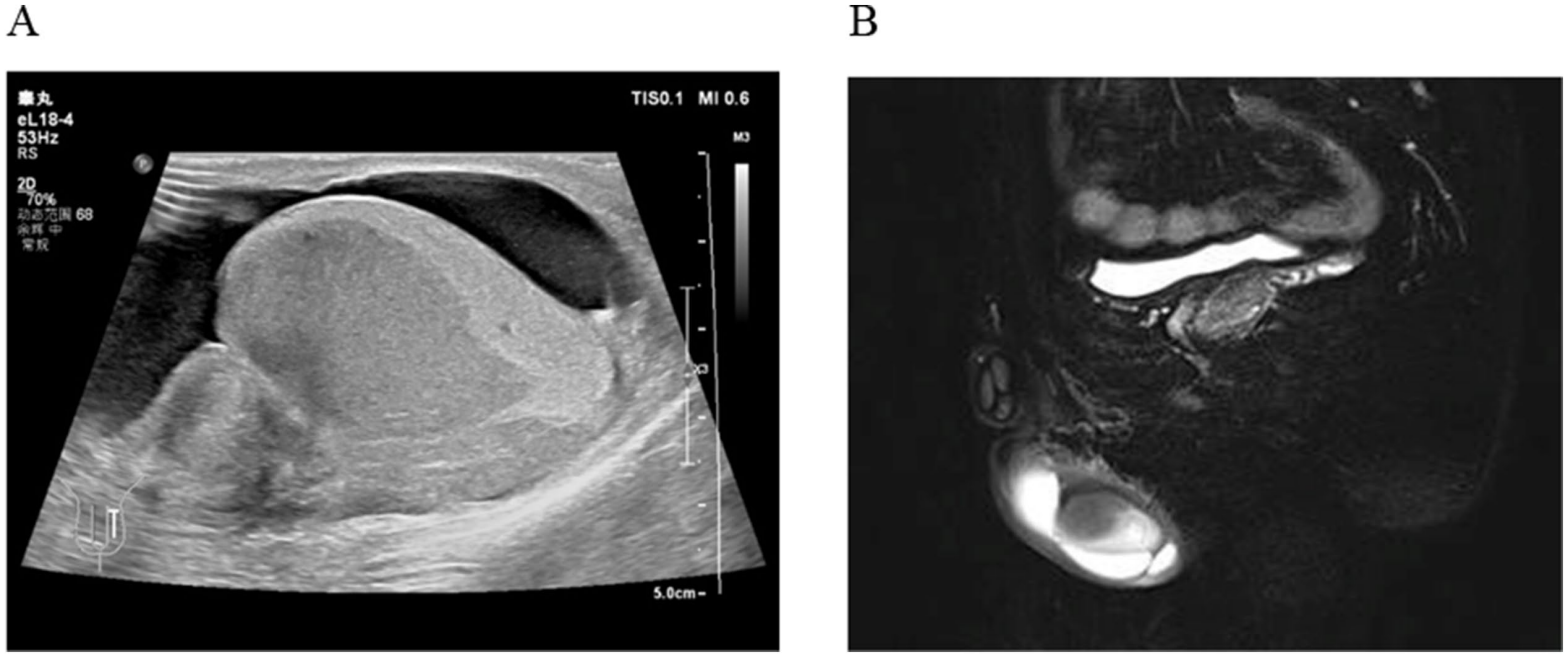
(A) Ultrasound shows a hypoechoic area in the left testicle; Enlarged left epididymis with abundant blood supply. (B) MR shows patchy abnormal signals in the left epididymis and testes.
